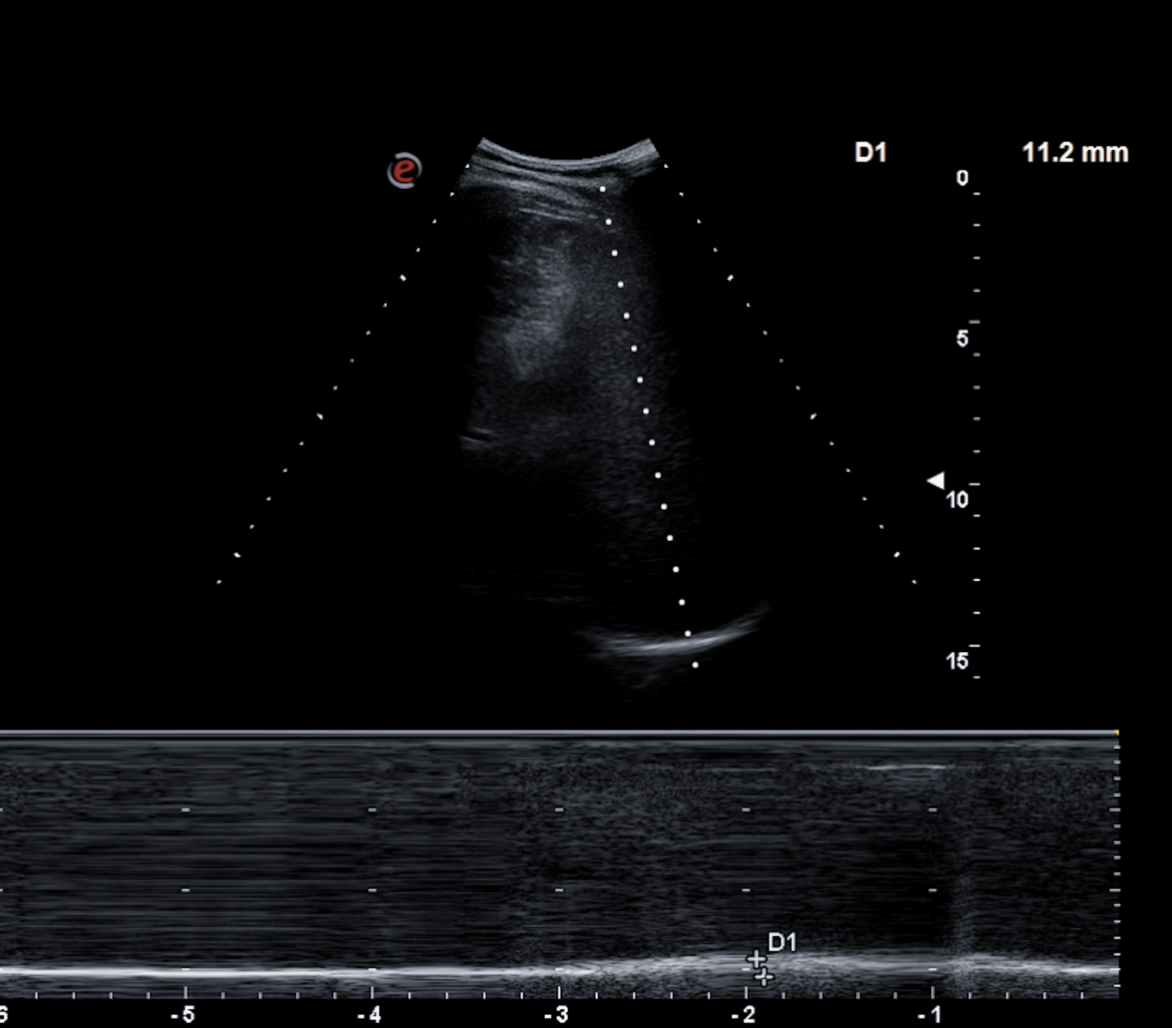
M-mode thoracic ultrasound showing reduced right hemidiaphragmatic excursion (<1 cm), indicative of partial right hemidiaphragmatic paralysis The two crosses in the bottom line labeled as D1 are very close indicating a distance of <1 cm
